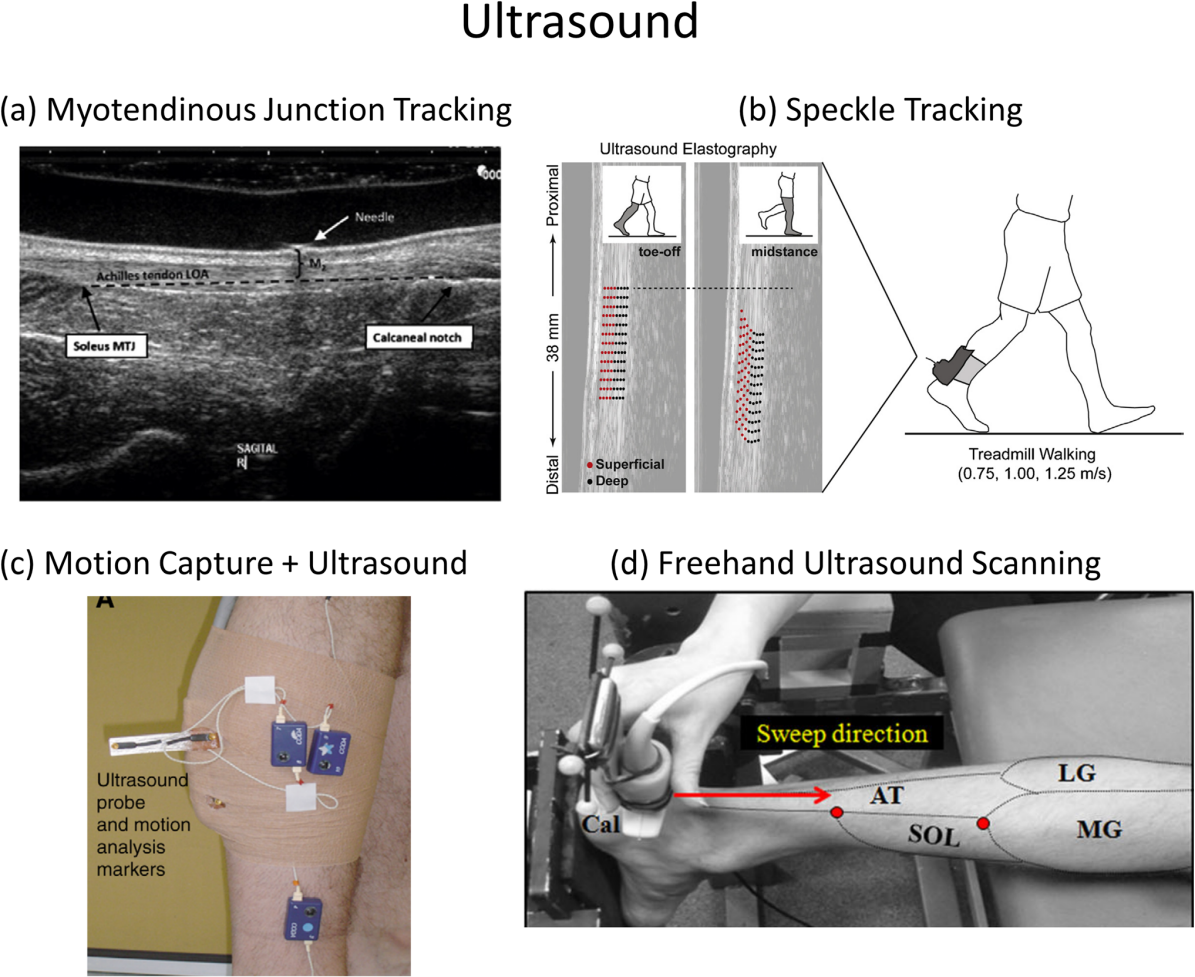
A variety of ultrasound techniques to quantify muscle and tendon elongations. (a) Ultrasound transducer with a wide FOV (100 mm) can image the entire free Achilles tendon (soleus to calcaneus). (b) The ultrasound elastography speckle tracking method enables the displacements of the superficial and deep AT tissues to independently measured during walking. (c) By fixing motion capture markers to the ultrasound transducer, tissue displacements in the sATMJ can be tracked in the laboratory coordinate system to quantify AT length changes. (d) An ultrasound transducer with markers attached is manually swept along the AT to take a series of ultrasound images of the AT. The ultrasound images and transducer positions are merged to reconstruct 3D geometries of the AT at multiple poses. (Included figures are adapted from Ref. 50, 94, 105, and 125).
